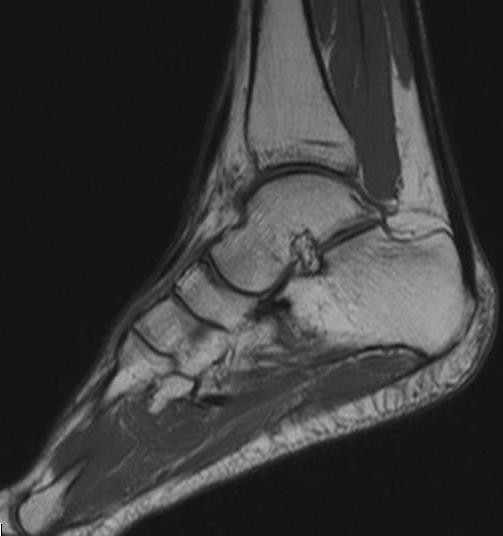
An image of the follow-up MRI of the right ankle 24 months after surgery. A sagittal T1-weighted spin-echo MR image showing subcutaneous scars anterior to the ankle.
